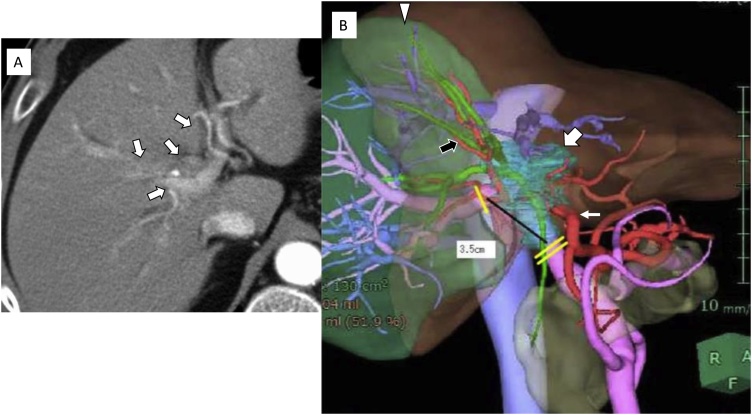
Contrast-enhanced CT showed an extensive PC, involving the middle and right hepatic arteries (white arrow); however, the posterior branch of the hepatic artery had not been invaded (a). Three-dimensional surgical planning images and fusion images composed of the planning images and cholangiographic images showed locally advanced PC, involving bile ducts, hepatic arteries, and the portal vein (the trunk and bilateral branches) (the blue area indicated by the thick white arrow) (b). The tumor had also invaded the anterior sectional bile duct as well as the anterior sectional hepatic artery and portal vein (black arrow), but the posterior equivalents of these structures had not been affected by the tumor. The proper hepatic artery had not been invaded by the tumor (thin white arrow). The volume of the future remnant liver (green area) was predicted to be 48% of the original liver volume.
